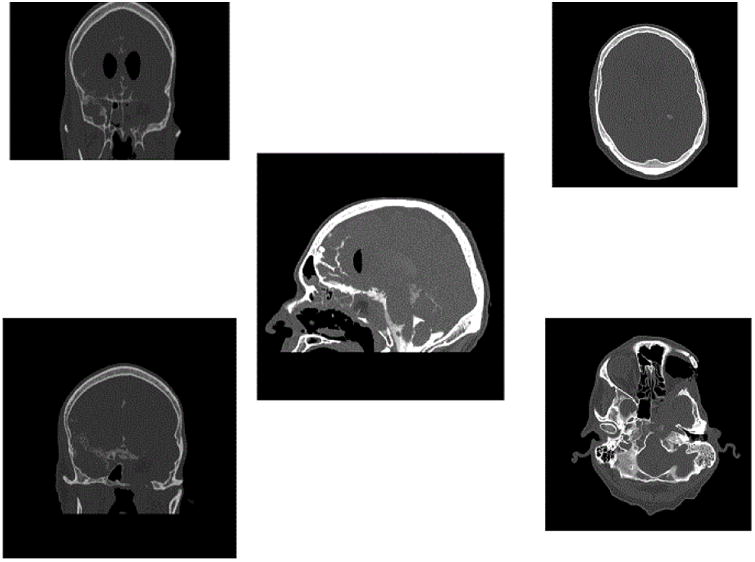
Post-operative CT cisternogram showing no CSF leak
